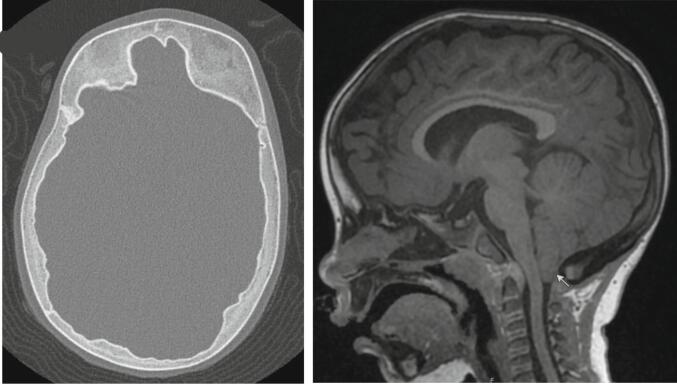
Computed tomography (CT) and magnetic resonance images (MRI) performed at age 15 months old. CT revealed fusion of the coronal sutures and MRI showed the presence of Chiari malformation type 1 (marked by a white arrow).
